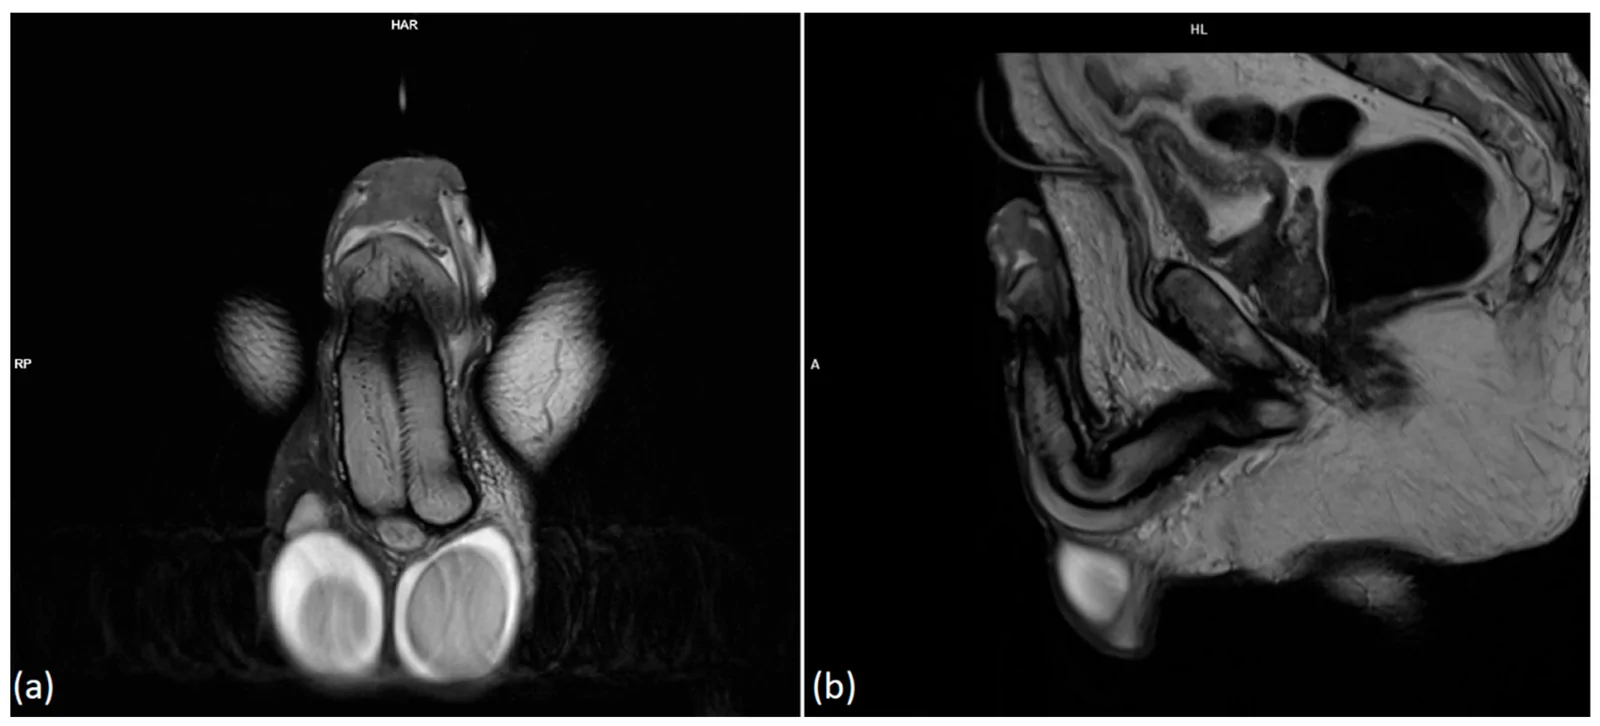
Tumour confined to the epithelium or subepithelial tissue of the glans without radiological evidence of corpus spongiosum or corpus cavernosum invasion (≤T1): (a) axial and (b) sagittal T2-weighted images.
